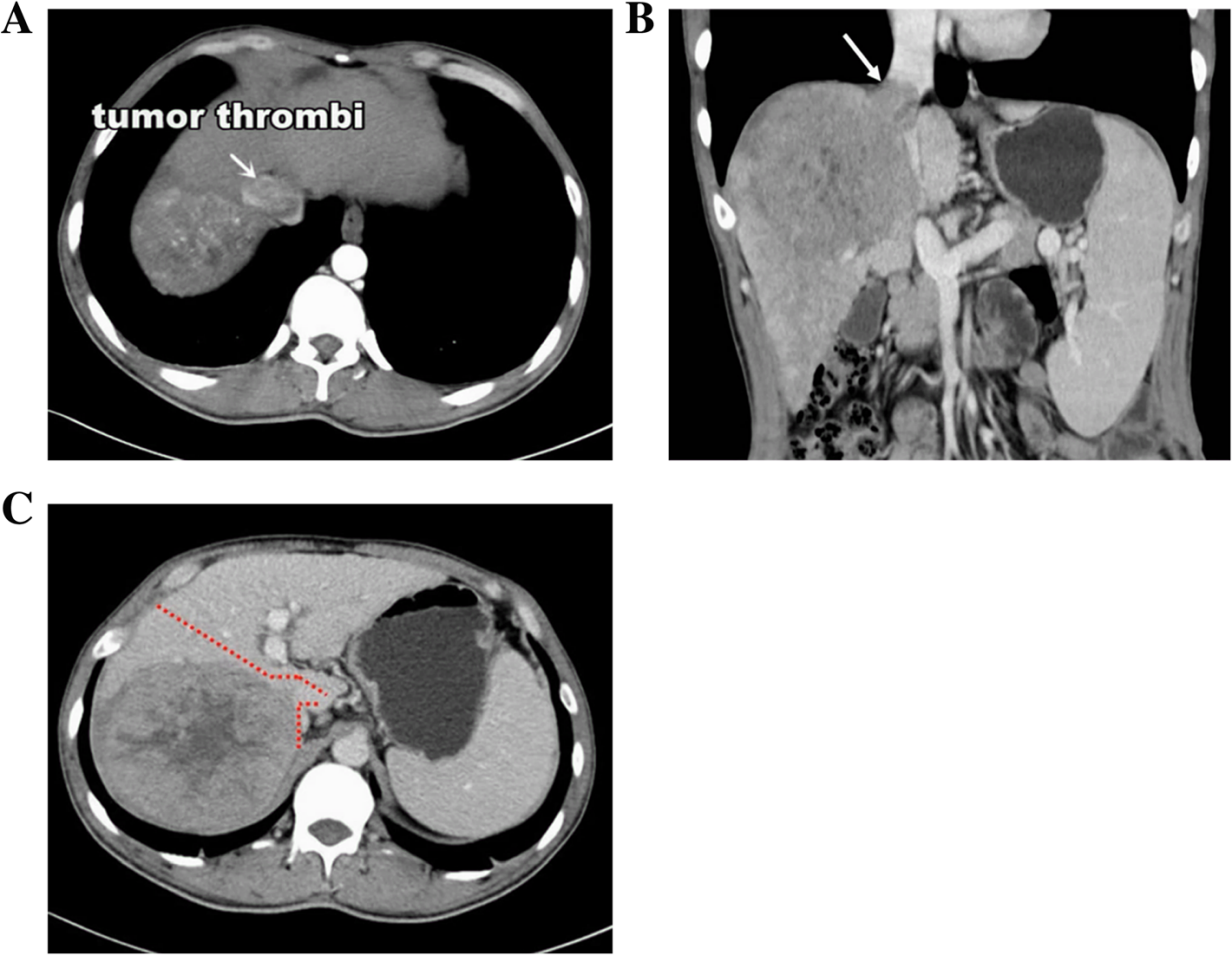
a CT scan showing a huge HCC located in the right hepatic lobe with tumor thrombus (arrow) entering the IVC. b Coronal CT suggests IVTT had passed the diaphragm level, but had not enter the right atrium (arrow). c Anterior approach right hepatectomy combined with IVCTT thrombectomy
